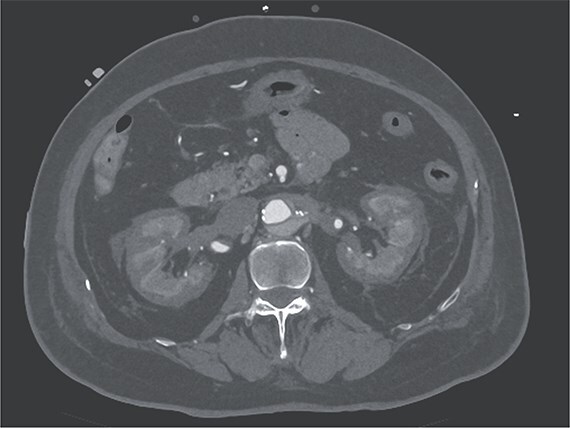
Contrast-enhanced CT image acquired on postoperative Day 2, showing the reverse rim sign.
